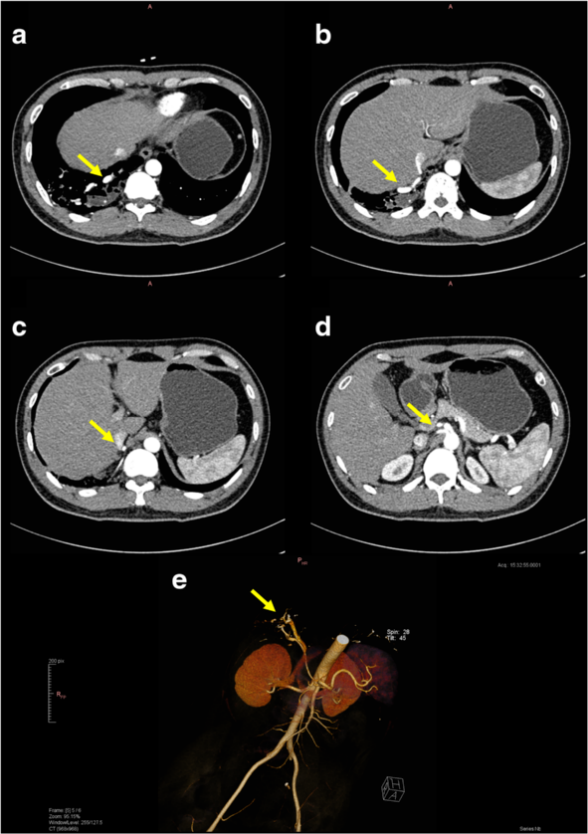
CT scanning with IV contrast confirmed the diagnosis of right lower lobe pulmonary sequestration by clearly demonstrating the aberrant feeding vessels (*yellow arrows*). a-d The entire course of the vessels from the abdominal aorta to the right lower lobe can be traced. e Detailed visualization from 3D reconstruction facilitates pre-operative planning
